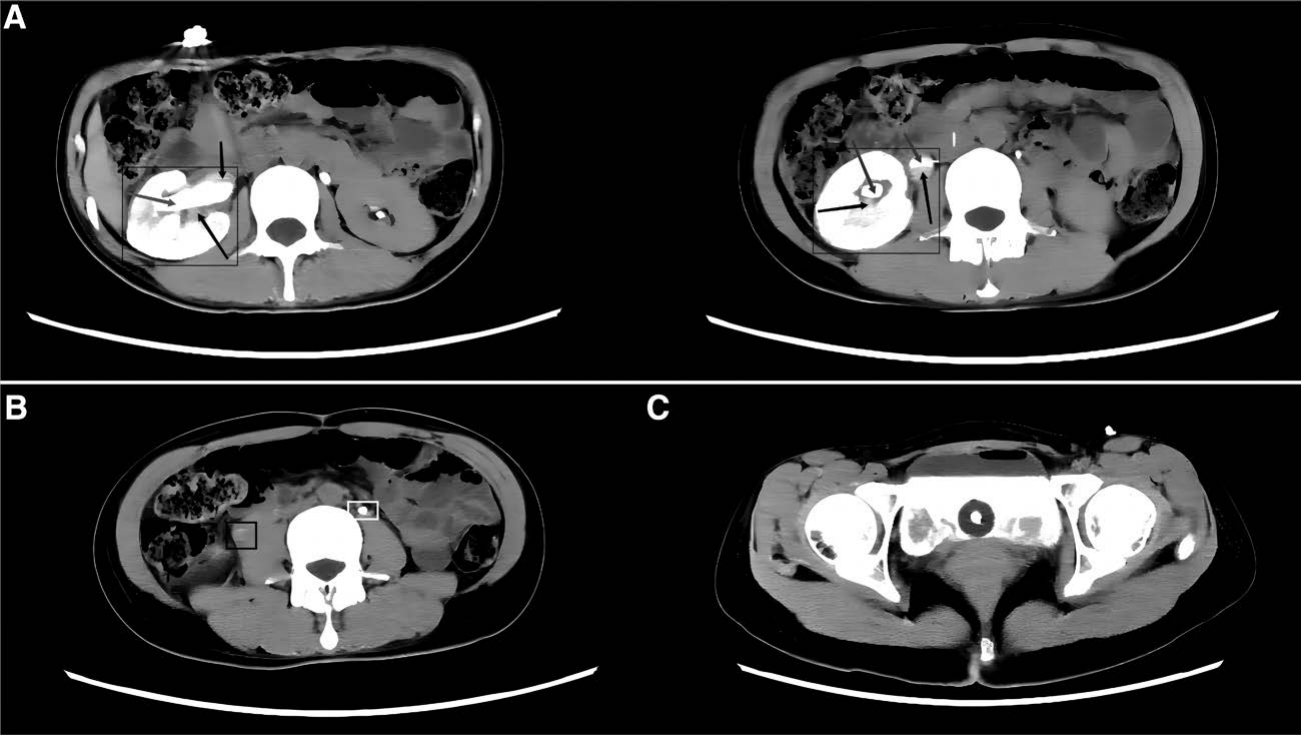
Computed tomography angiography. (A) Compared to the left side, the right renal pelvis and upper middle ureter are dilated and strips of white high-density shadow (gray arrow in black quadrant) and some gray low-density (black arrow in black quadrant) can be seen. (B) Compared to the left ureter (white quadrant), only a small amount of contrast filling is seen in this segment of the right ureter, and the lumen is mostly gray hypodense shadow. (black quadrant). (C) A large number of white high-density shadow and gray low-density shadow in the bladder can be seen.
